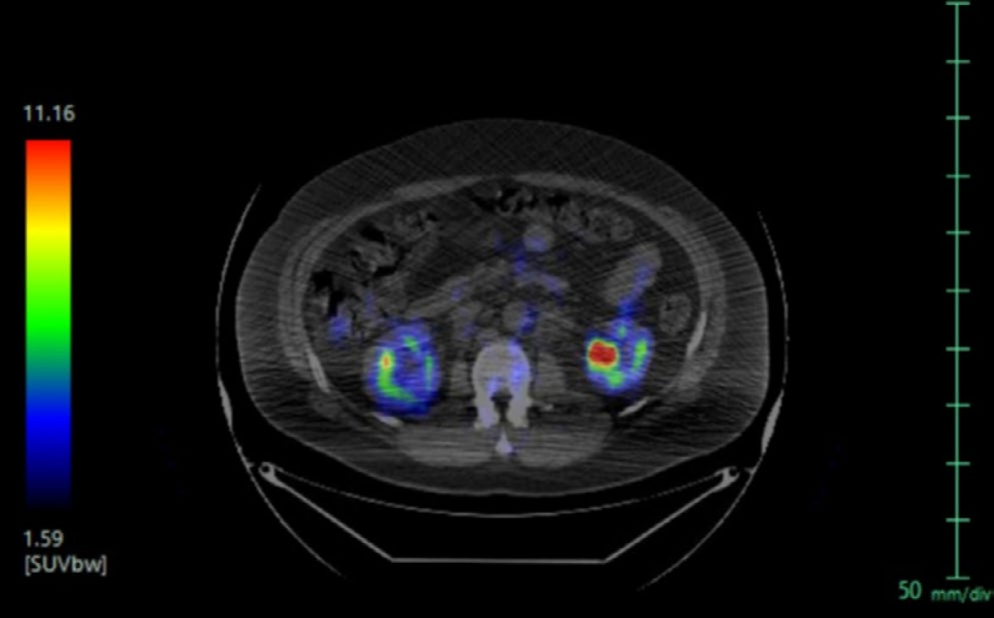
CT‐PET scan of the skull base to mid‐thigh obtained after the sixth and final cycle of R‐CHOP showed improved retroperitoneal adenopathy. The lymph nodes were FDG avid, suggestive of residual disease
